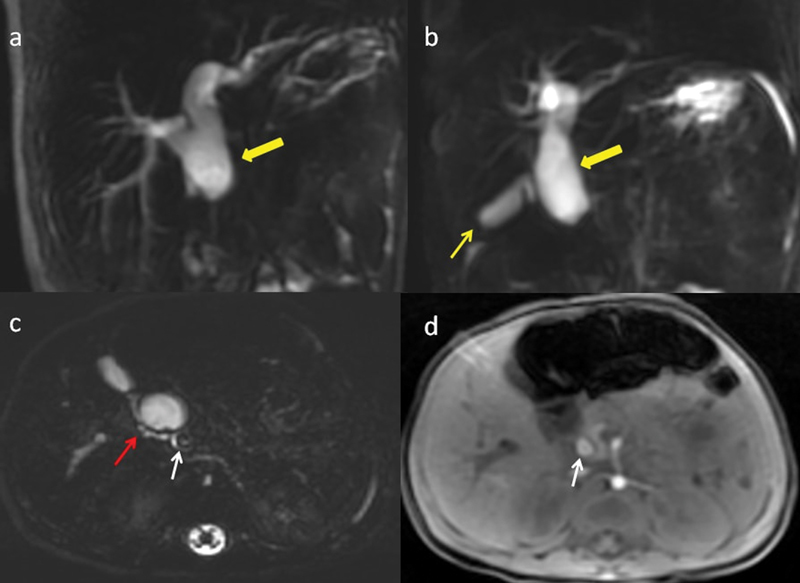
MRI images of a 3-month-old infant with cholestatic jaundice. (
